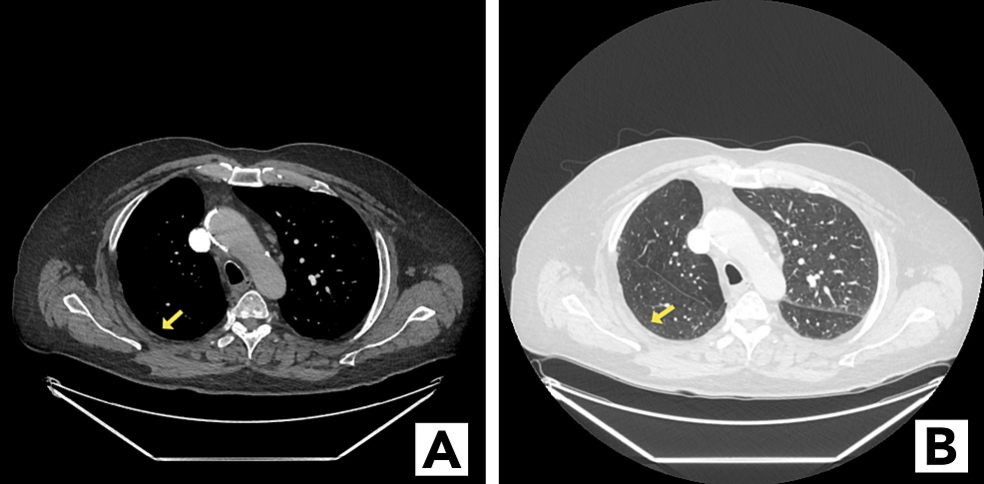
CT images six months later showing complete resolution of nodular subpleural mass in the right lower lobe (arrow). (A) Mediastinal window; (B) lung window
